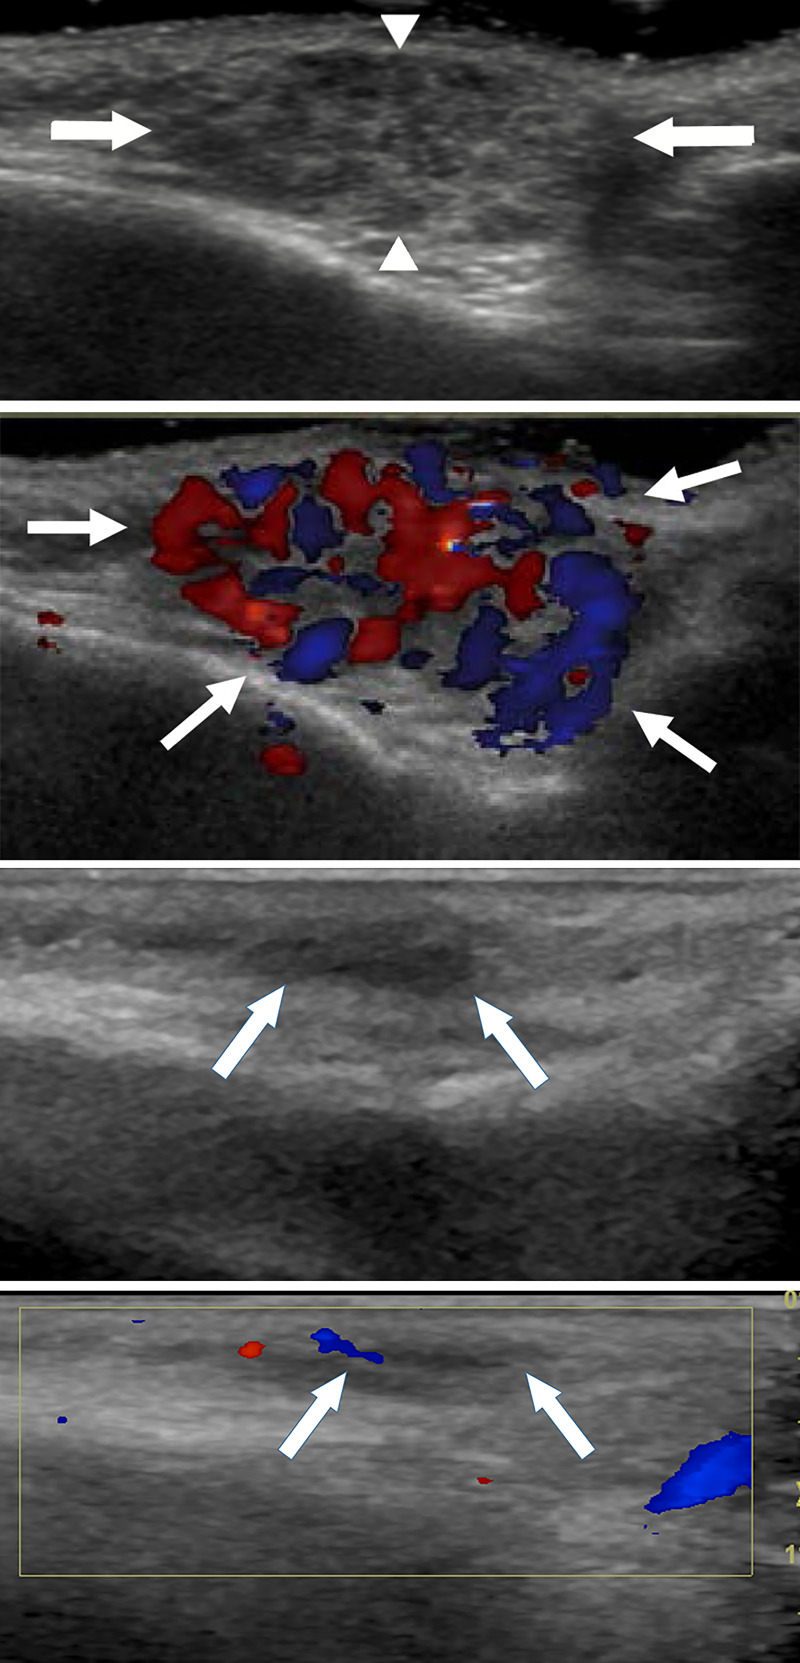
Image of a 4-month-old girl with infantile hemangioma who had near complete regression after 1 year of propranolol treatment. (a) Initial gray-scale US image shows a hypoechoic subcutaneous mass (arrows) without deep tissue involvement. Lesion length was defined as the longest diameter of the tumor (distance between arrows). Lesion height was defined as the longest distance between superficial and deep margins of the lesion (distance between arrowheads). (b) Initial color Doppler image depicts hypervascularity within the lesion (arrows). (c) Follow-up gray-scale US image shows decreased size of the lesion (arrows) (d) Follow-up color Doppler image depicts hypovascularity within the lesion (arrows), showing interval decreased vascularity.
